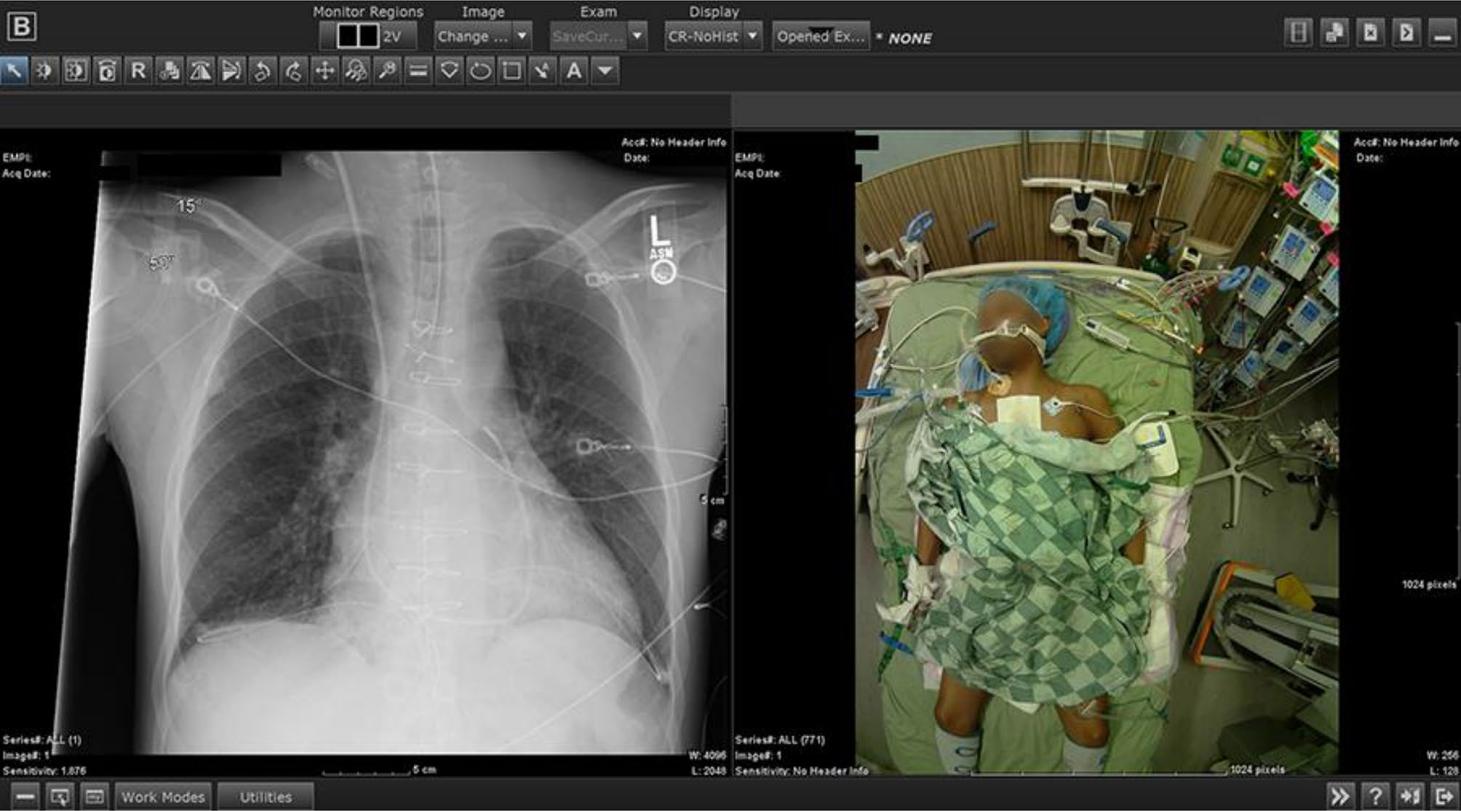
Portable radiograph along with the simultaneously acquired point-of-care photograph displayed at a PACS workstation
